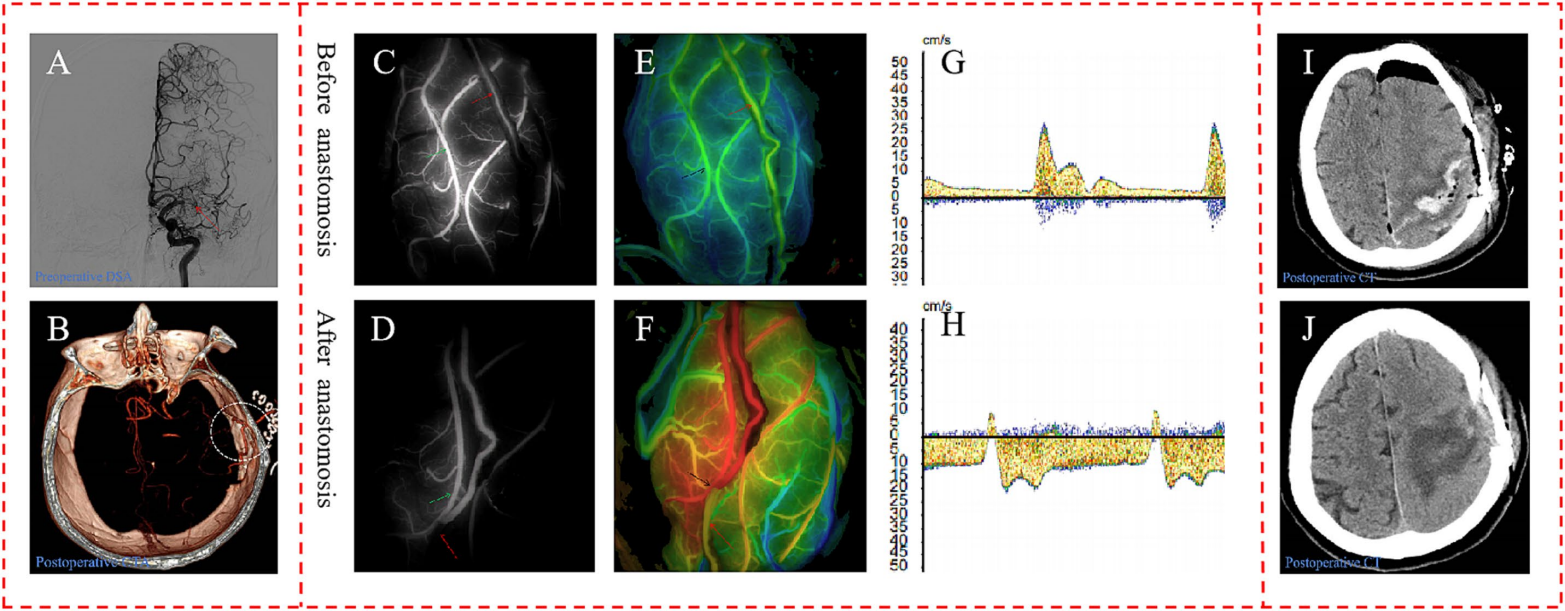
Representative case. (A) Preoperative DSA showed initial segment occlusion of the left MCA with abnormal smoke-like vessels (red arrow). (B) Postoperative CTA confirmed the patency of the bypass, with the anastomotic stoma highlighted within a white circle. The ICG-VA demonstrated the patency of the bypass and a delay in the distal donor vessel, in which the donor vessel was indicated by the red arrow, and the recipient vessel is marked with a green arrow (C,D). In the Flow800 imaging, there was an abrupt change in the color of the distal STA when compared to the preoperative state, as indicated by the red arrow (E,F). And MDU showed the direction of distal STA was bidirectional, compared to the unidirectional flow observed preoperatively (G,H). The initial postoperative CT scan on the first day after surgery displayed evidence of cerebral hemorrhage (I). By the second week post-operation, the hematoma had been nearly entirely absorbed, indicating a recovery in the patient’s condition (J).
